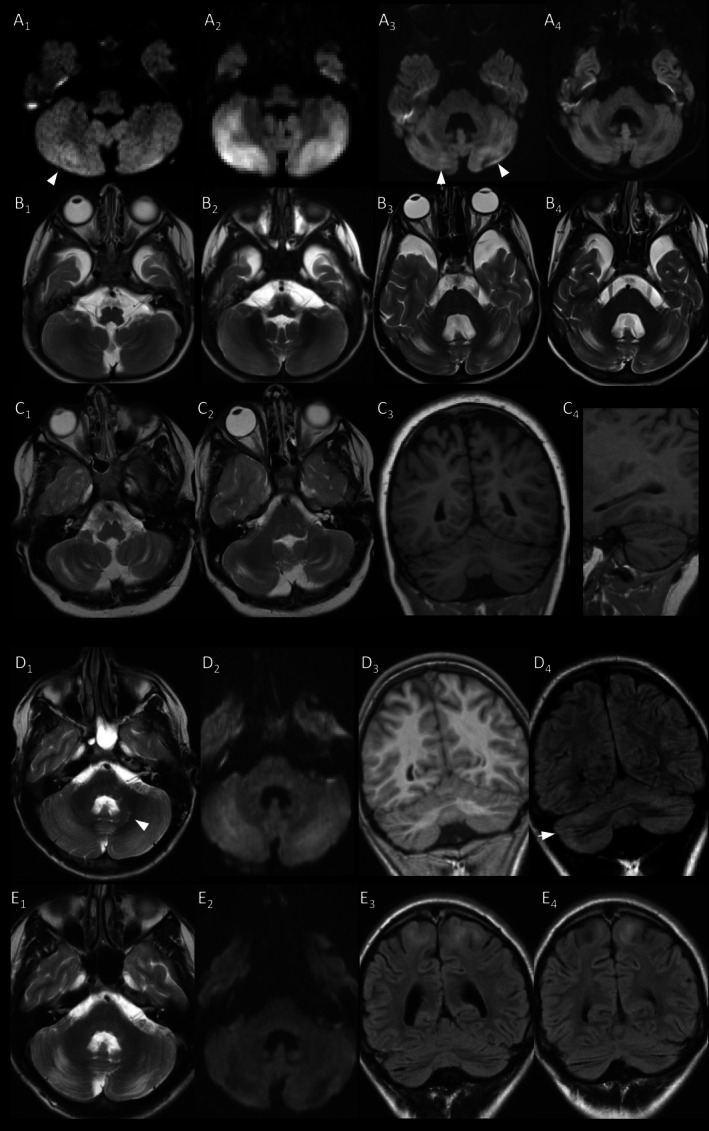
Examples of cerebellar watershed injury. (A, B) Evolution of cerebellar watershed injury in p4 with mild, focal hyperintensity in the right cerebellar hemisphere on diffusion‐weighted image (A_1_) at 1.1 years during acute metabolic decompensation. Hyperintensity is bilateral and more extensive at 1.9 years during another acute episode (A_2_), while T2‐hyperintensity is difficult to appreciate (B_2_). Atrophy with widening of depth of cerebellar fissures has developed by 12.1 years a further acute episode (B_3_). Note the small adjacent areas of increased signal on DWI consistent with acute injury (A_3_), which have resolved on follow‐up at 12.6 years (A_4_; compare with signal of temporal cortex). (C) Atrophy of cerebellar watershed areas in p10 at first MRI at 9.8 years during acute metabolic decompensation with characteristic involvement of depth of cerebellar fissures. (D, E) Combination of focal atrophy consistent with pre‐existing injury (D_4_, arrowhead) and more extensive (sub)acute cerebellar watershed injury with T2/FLAIR/DWI‐hyperintensity and mild T1‐hypointensity in p11 at first MRI at 15.4 years. NB T2‐hyperintense signal of dentate nucleus (arrowhead in D_1_). On follow‐up at 18 years signal changes have been superseded by atrophy. NB new changes of subcortical cerebral WM (E_3,4_), see also Figure 6. (DWI: A_1–4_, D_2_, E_2_; T2w: B_1–4_, C_1,2_, D_1_, E_1_; T1w: C_3–4_, D_3_; FLAIR: D_4_, E_3,4_.)
